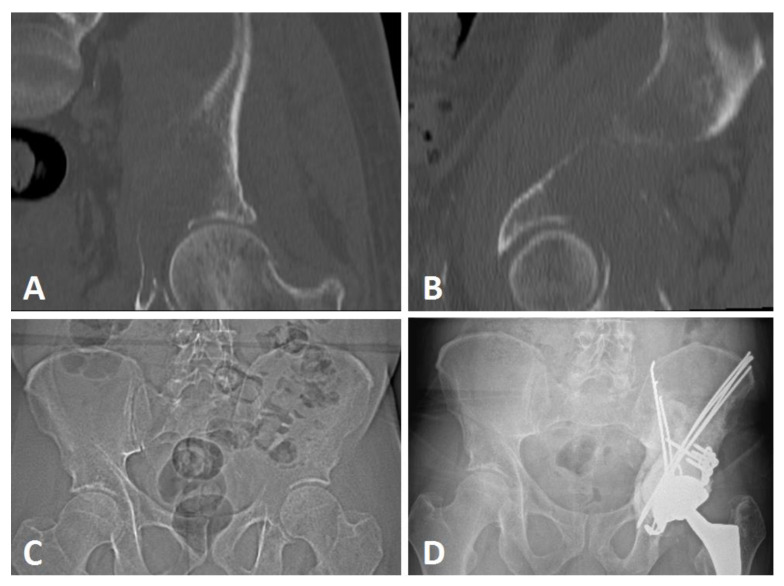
Imaging views of a renal clear cell carcinoma metastatic iliac lesion Harrington class III. Frontal view (A) of the periacetabular lesion, sagittal view (B), X-ray AP view of the pelvis before surgery (C), and postoperative X-ray (D) at 22-month follow-up.
